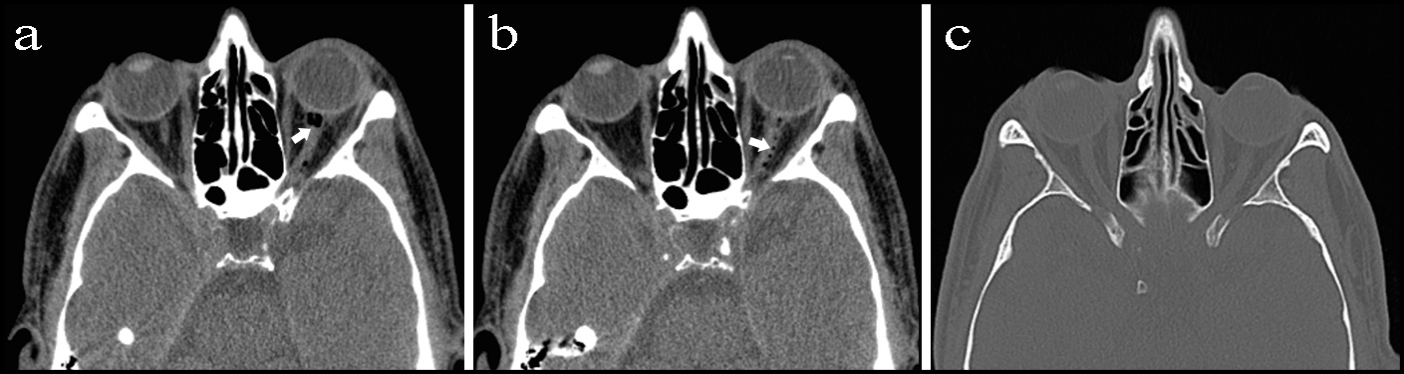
Computerized tomography (CT) images of the optic nerve sheath penetration (a) Air bubbles within the left (operated) optic nerve indicate optic nerve sheath penetration (arrow). (b) Movements of air bubbles to the chiasm (arrow). (c) The follow-up CT scan at day 3 shows disappeared air bubbles in the left optic nerve sheath.
